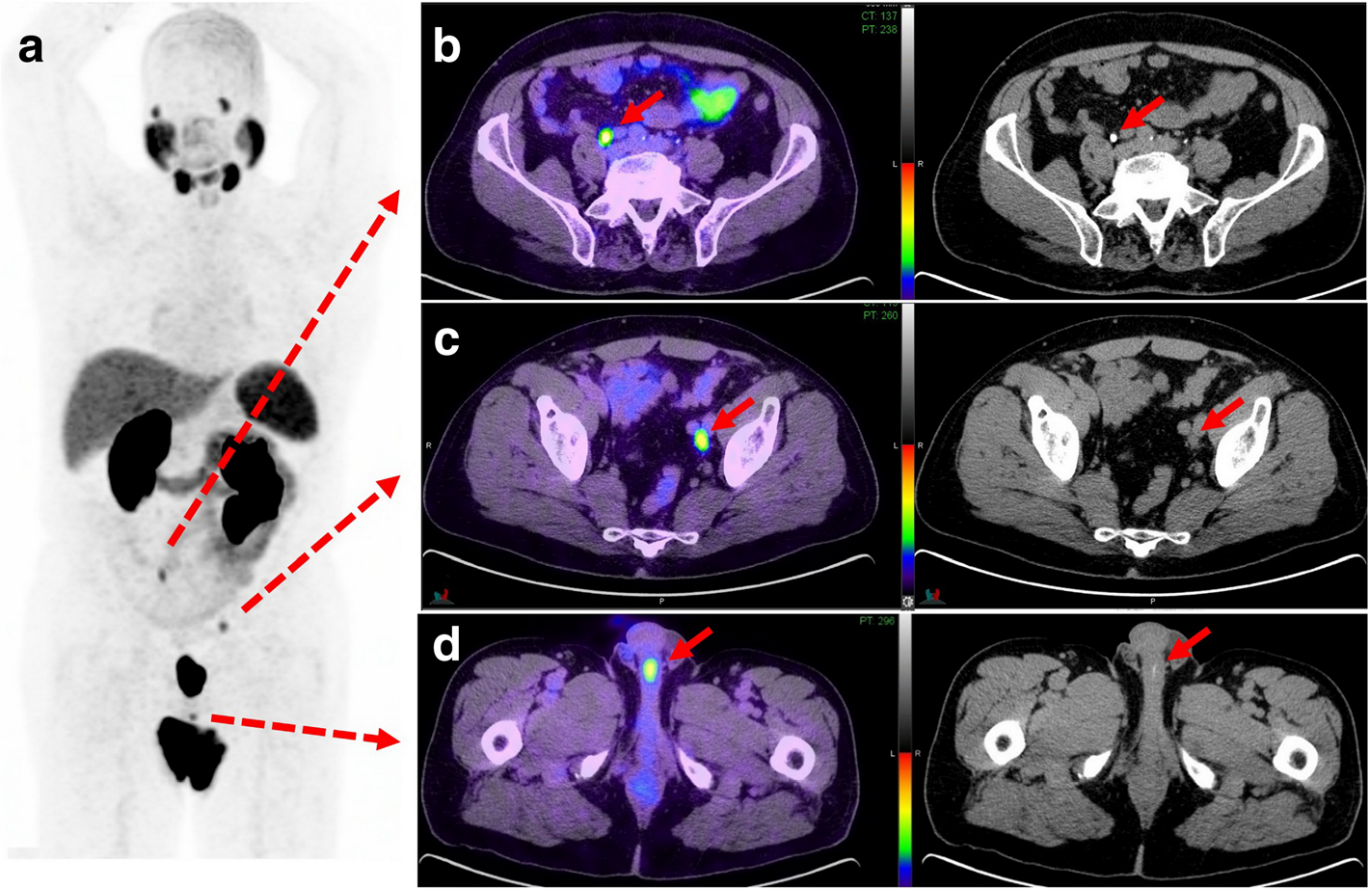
Sixty-six-year-old patient with BCR and PSA of 1.5 ng/L. Panel a. PSMA PET maximum intensity projection (MIP) image shows three foci of activity. Panel b. PET/CT-U and CT-U axial images shows focal activity corresponding to opacified right ureter (arrows). Panel c. PET/CT-U and CT-U axial images show focal uptake corresponding to a left pelvic lymph node (arrows). Absence of urinary opacification excluded the urinary pooling as potential explanation for this focal activity and this focus was reported as nodal metastasis. Panel d. PET/CT-U and CT-U axial images show focal activity corresponding to contrast agent in the urethra (arrows)
